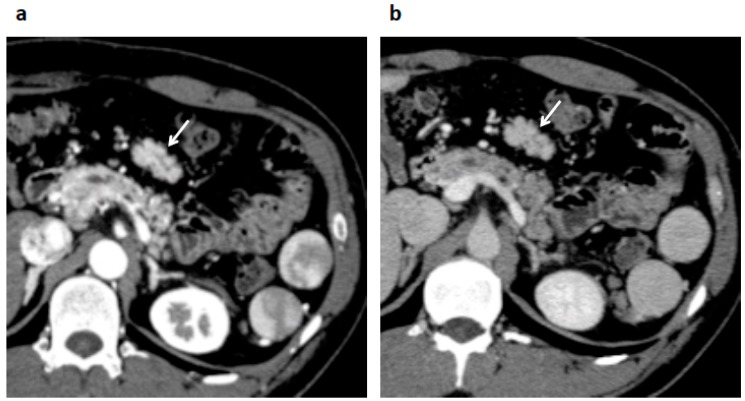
Computed tomography (CT) showing spreading original spleen tissue and an enhanced multinodular lesion (arrow) located anterior to the pancreas body, 35 mm in size, with a vascularity level similar to that of the original spleen at 40 s (a) and 70 s (b) after contrast injection.
